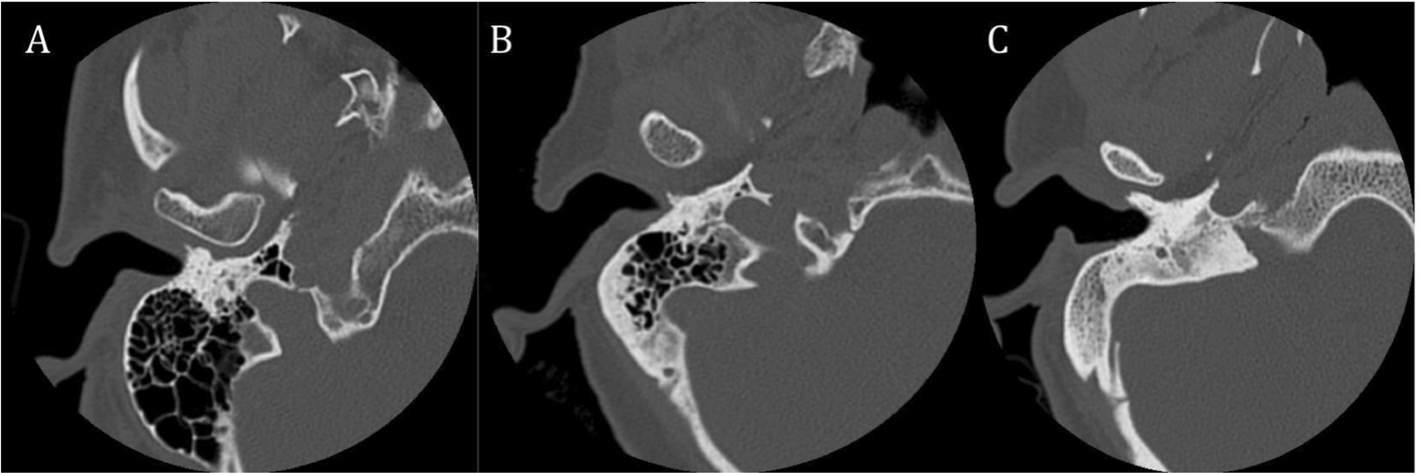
Representative axial images of pre-operative high-resolution temporal bone CT scan showing mastoid that is (a) well aerated, (b) moderately aerated, and (c) poorly aerated
